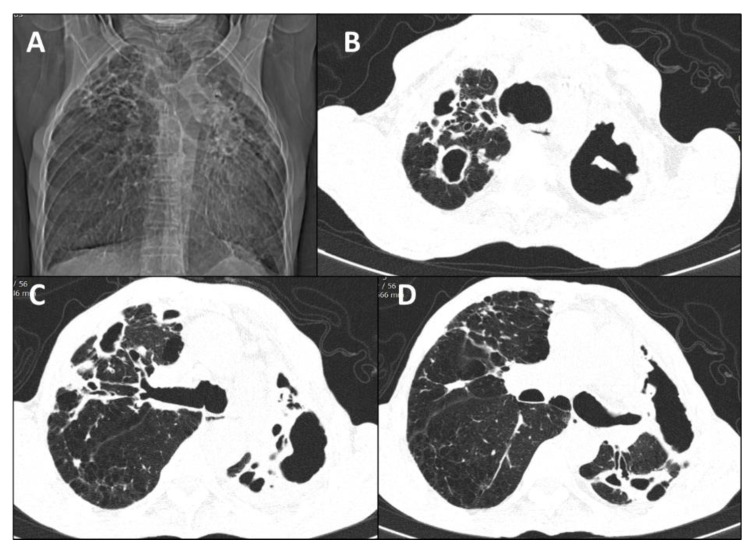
Primary pulmonary sporotrichosis in a 71-year-old man (Case 1). (A) Topogram depicts lung cavitary lesions. (B–D) Axial nonenhanced chest computed tomography images show extensive thick-walled cavities with irregular margins in upper lobes. The cavitary lesion is more predominant in the left upper lobe, associated with thick septations and pulmonary volume loss.
